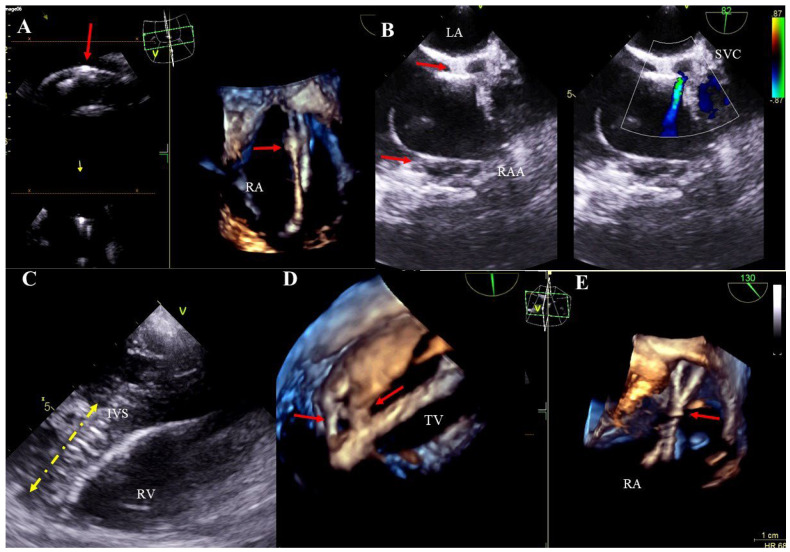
TEE (2D, 3D) showing scar tissue around the leads. Segmental thickening of the leads and lead-on-lead adhesions in the right atrium (red arrow) (A). Pathological attachment of the two leads to the interatrial septum and to the atrial wall near the atrial appendage (red arrows). The narrowing of the vena cava at entry into the atrium is caused by the thickened leads and pathological scar tissue (Doppler color) (B). Thickened ventricular lead (yellow line) pathologically attaches to the endocardium of the interventricular septum in the right ventricle (C). The image from the right ventricle depicts a pathological adhesion (red arrows) of the lead to the edge of the tricuspid valve leaflet (D). Binding and intersection of thickened leads in the atrium (red arrow) (E).
